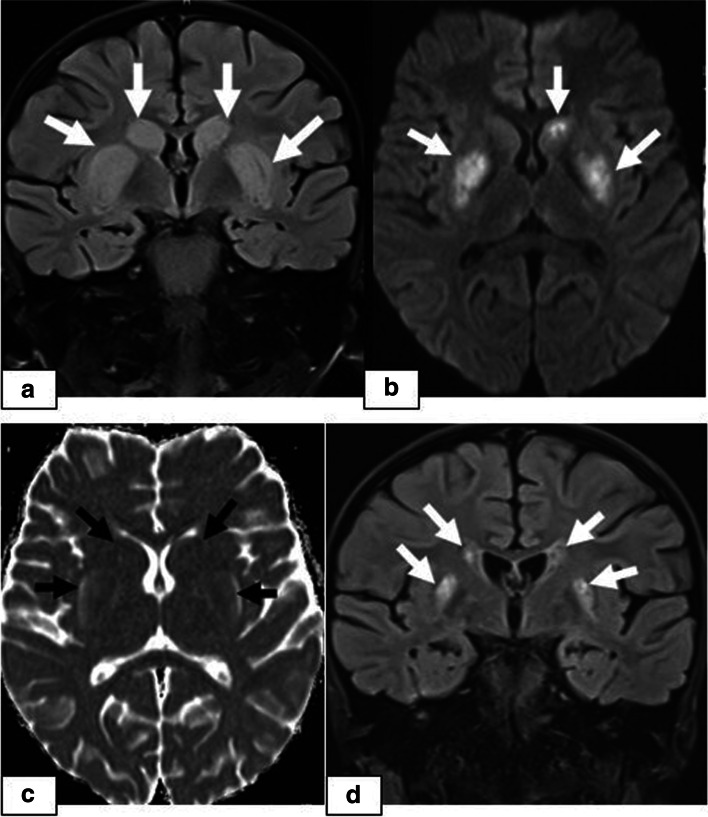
A 17-year-old girl presenting with an altered level of consciousness 6 months after undergoing allogeneic HSCT for thalassemia major. a Coronal FLAIR image showing hyperintensity in the bilateral basal ganglia (arrows). b DWI showing increased signal intensity in the basal ganglia. c ADC map showing normal signal intensity, which was considered to be indicative of ADC pseudonormalization. d Follow-up coronal FLAIR image after 6 months demonstrating persistent hyperintensity associated with volume loss, suggesting cytotoxic injury
